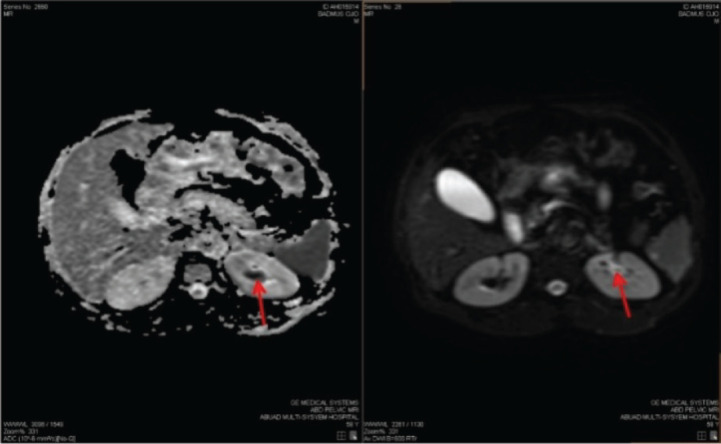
MRI. DWI and ADC images showing a focal area of diffusion restriction in the left upper pole calyx (red arrow).
